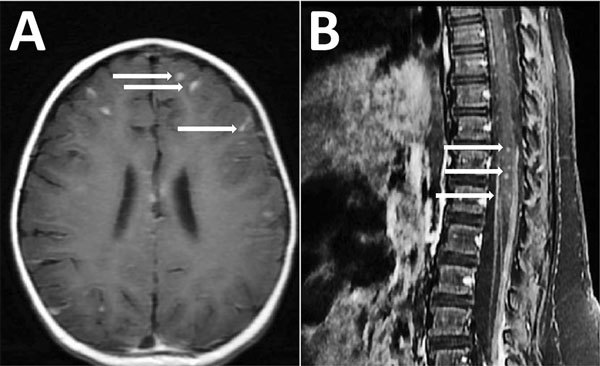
Magnetic resonance imaging (MRI) of the brain (A) and the spine (B) showing meningitis and myelitis in a 12-month-old girl with *Angiostrongylus cantonensis* infection, Houston, Texas, USA. A) Axial T1 post contrast sequences showing diffuse leptomeningeal enhancement (arrows). B) Sagittal T1 postcontrast sequences showing intramedullary enhancement in the thoracic and lumbar spinal cord T8–L5 with diffuse leptomeningeal enhancement (arrows).
